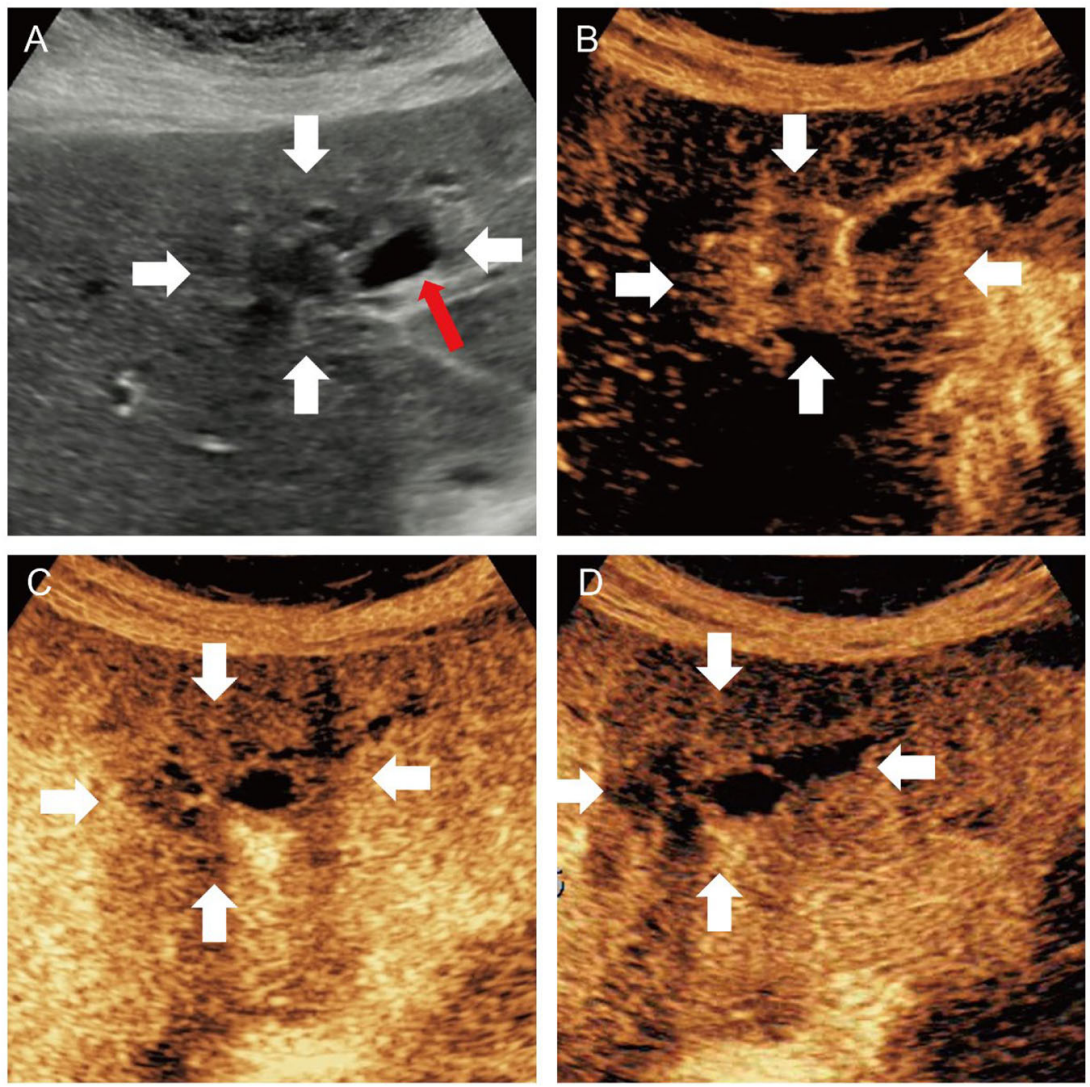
A 79-year-old female with a 14-mm biliary type MF-ICC. B-mode ultrasound showed hypo-echogenicity lesion and dilated bile duct (A). Circular enhancement was observed in the arterial phase (B). Hypo-enhancement was observed in the portal venous phase (C) and late phase (D). White arrow indicated the margin of the lesion; red arrow indicated the dilated bile duct
